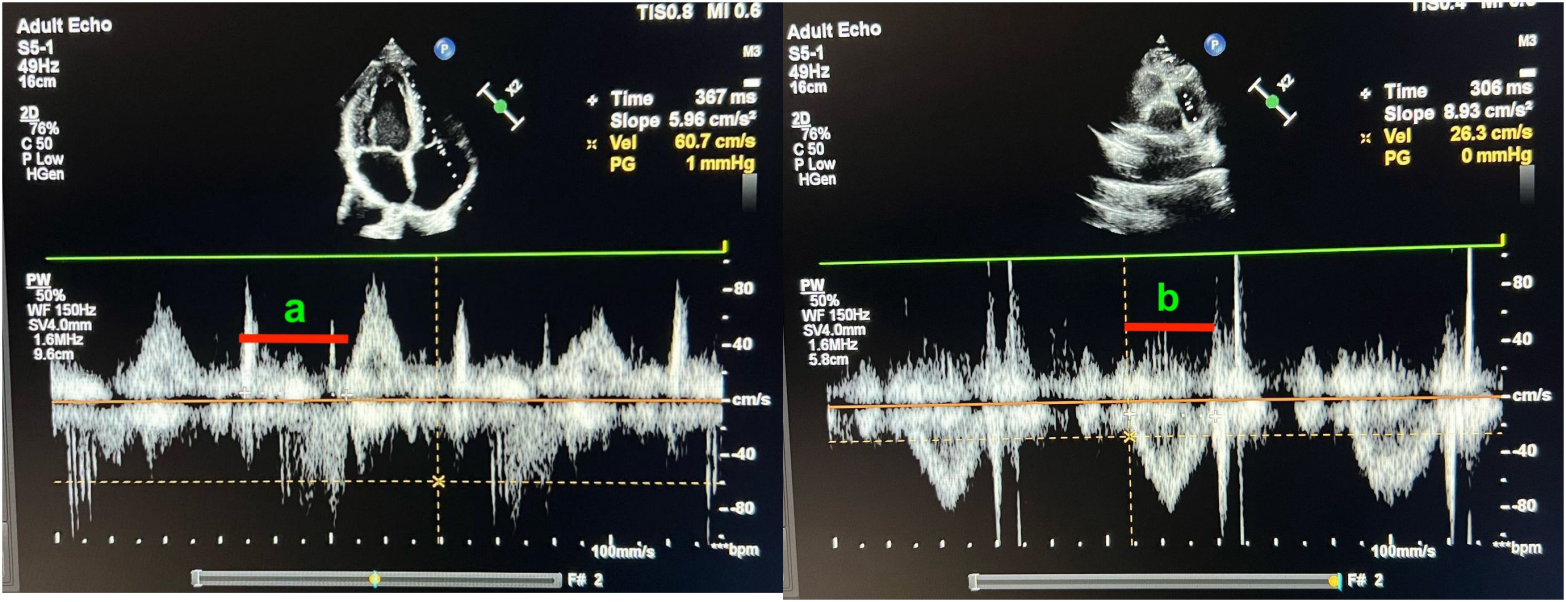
Measurement of right ventricular myocardial performance index (Tei Index) using pulsed-wave Doppler echocardiography. (a) Apical four-chamber view showing tricuspid inflow Doppler waveform. The interval labeled “a” corresponds to the total duration between tricuspid valve closure and opening (IVCT + ET + IVRT). (b) Parasternal short-axis view showing pulmonary outflow Doppler waveform. The interval labeled “b” indicates the ejection time (ET) of the right ventricle. The myocardial performance index (MPI) is calculated as (a - b)/b.
